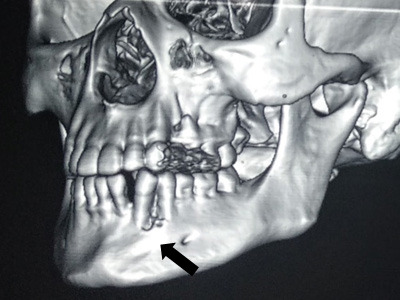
CT scan revealed a mild lytic lesion with an erosion of the alveolar cortex (indicated by a black arrow).
